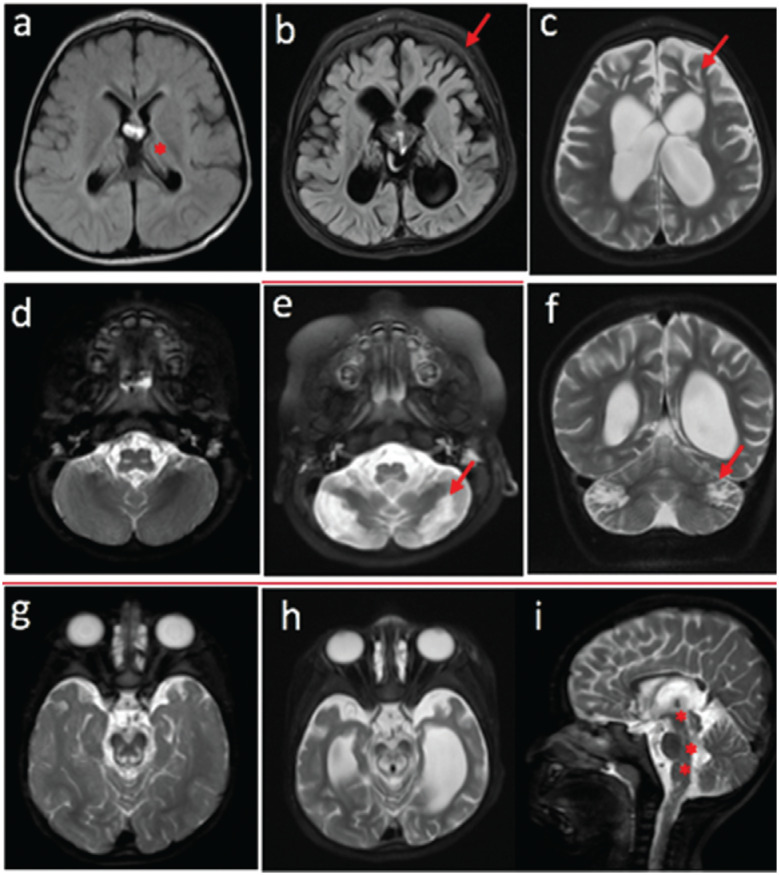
Brain magnetic resonance imaging (MRI). Axial flair sequence MRI at 2 years
